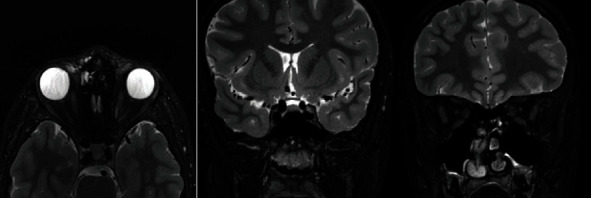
MRI T2 FAT-SAT sagittal and coronal images showing marked thinning of both optic nerves and also even if less of the chiasma and the optic tracts without any signal alteration.
